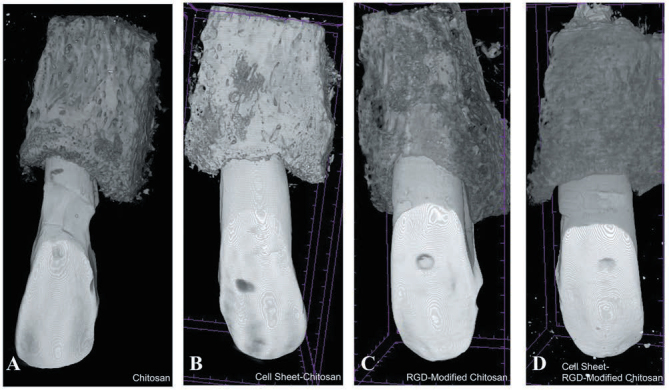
Micro-computed tomography scan analysis. (
